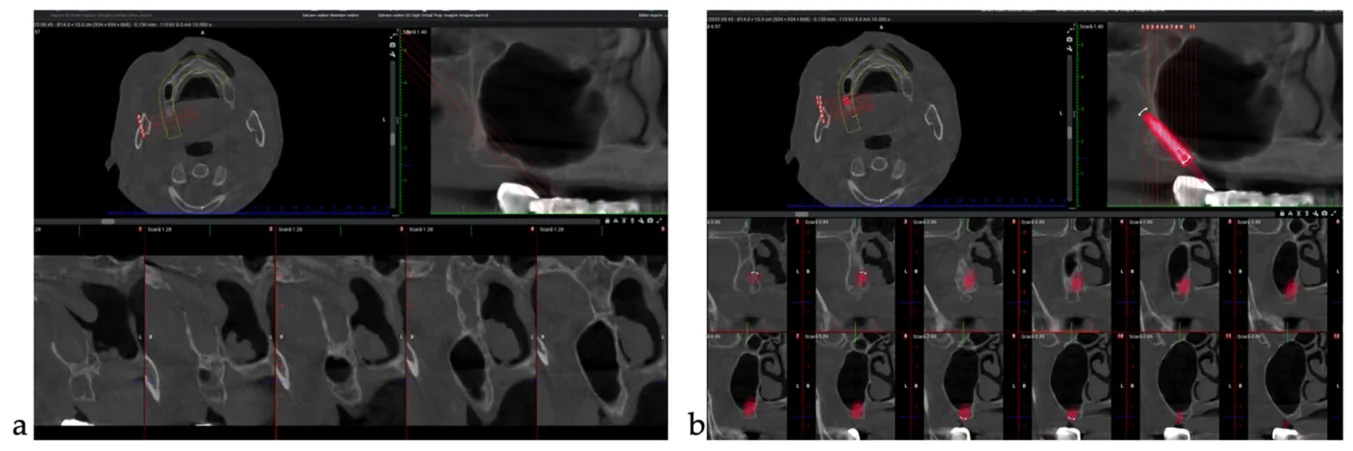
(a) Initial CBCT. (b) Pterygoid implant planning.
